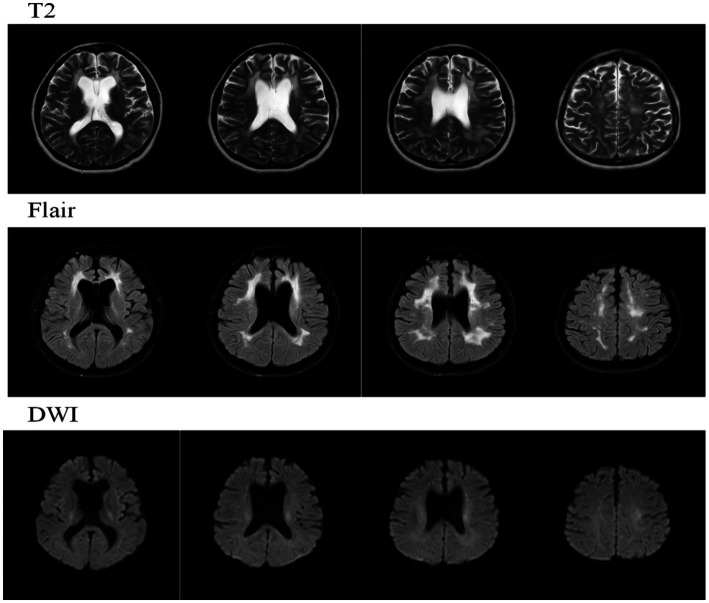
MRI and DWI findings on June 25, 2025.
